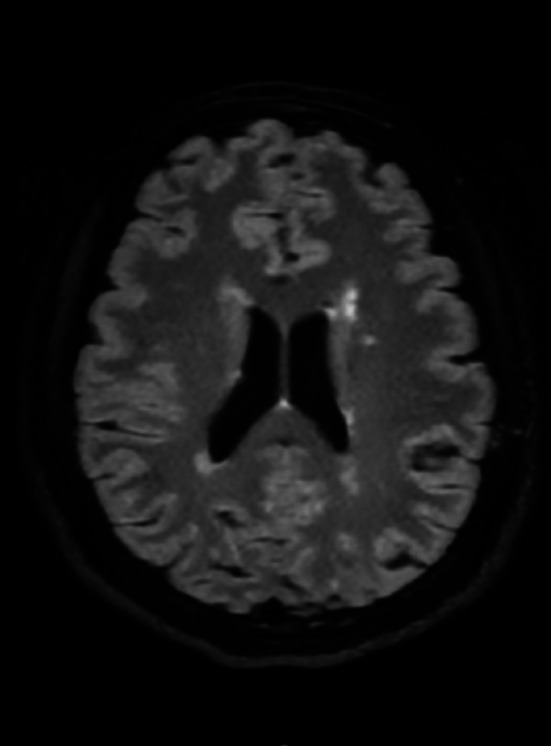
Brain MRI (T2‐FLAIR sequence) showing nonspecific white matter signal changes.
